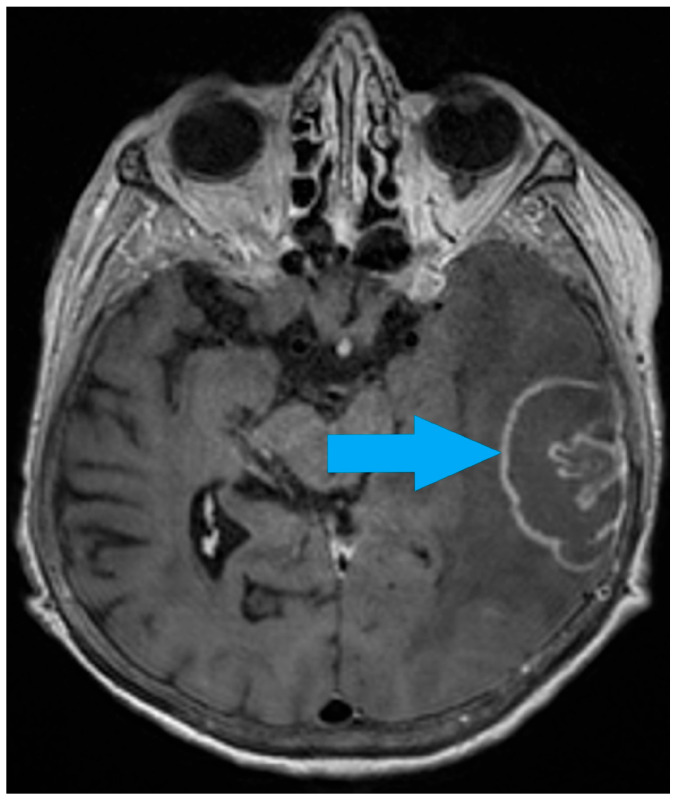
Magnetic resonance imaging (MRI)—a left temporoparietal expansile lesion (55 × 32 × 42 mm) with ring-enhancing post-contrast signal intensity—glioblastoma and features of intratumoral hemorrhage.
